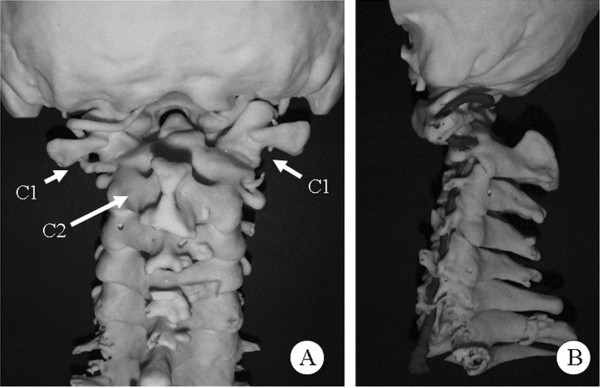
Stereoscopical model of the cervical spine based on three-dimensional computed tomography data. (A) The view from the posterior direction. (B) The view from the lateral direction. Complete absence of the C1 posterior arch and hypertrophy of the spinous process of the axis were observed.
